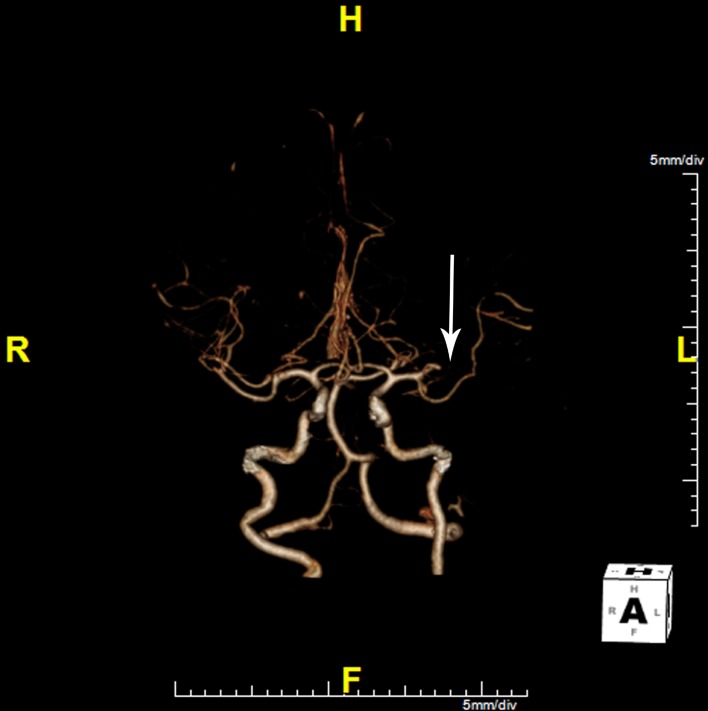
CT angiogram showing left MCA M2 occlusion (arrow).
